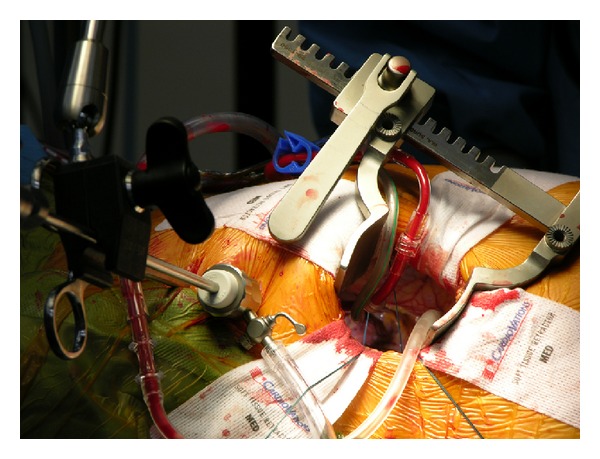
Direct transthoracic aortic clamping.
